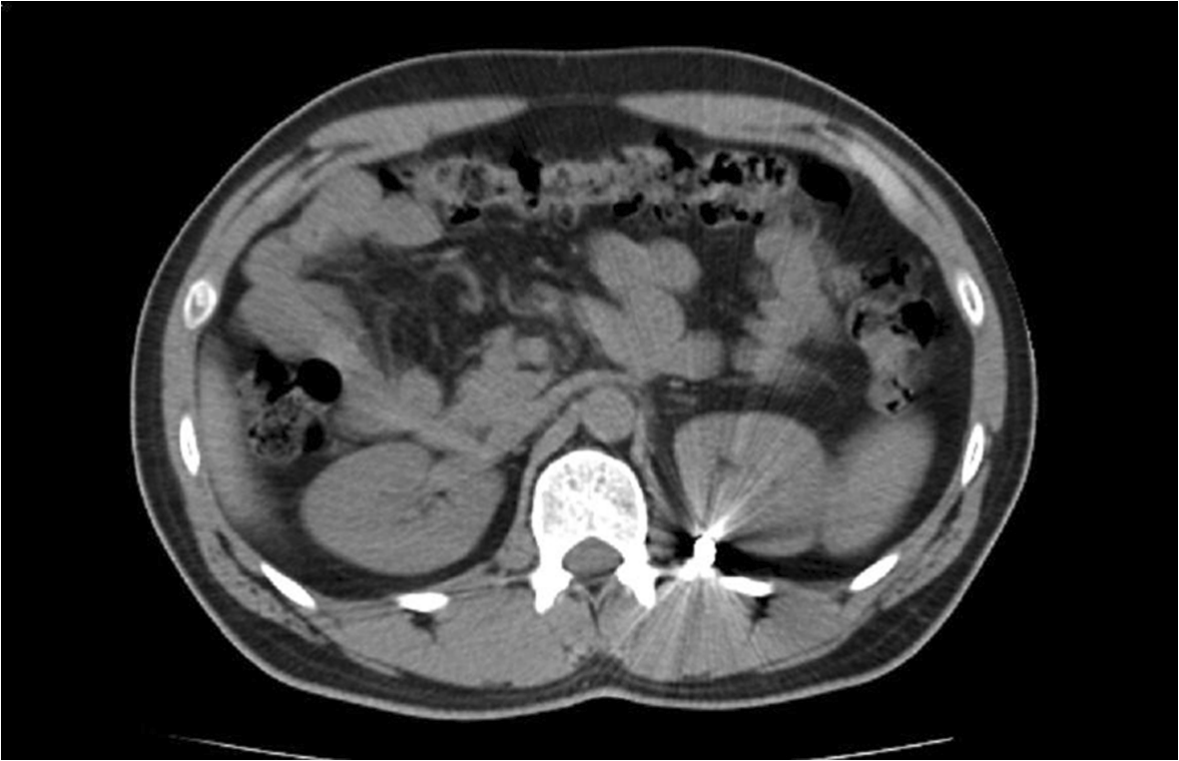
Transverse view of CT scan. This view shows the bullet to be lodged postero medially to the left kidney.
